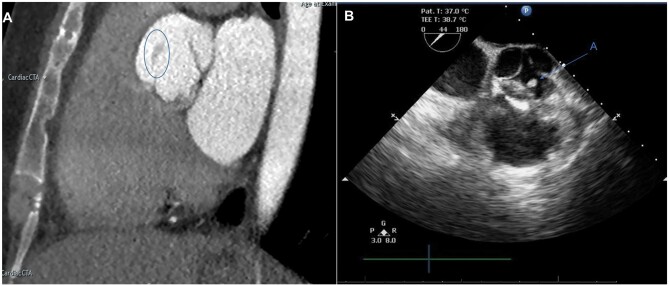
Gated CT of aortic valve. (A) Coronal view showing 7-mm lesion (blue outline). (B) Intra-operative transoesophageal echocardiogram. Short-axis parasternal view showing 7-mm ovoid lesion on right coronary cusp (A).
